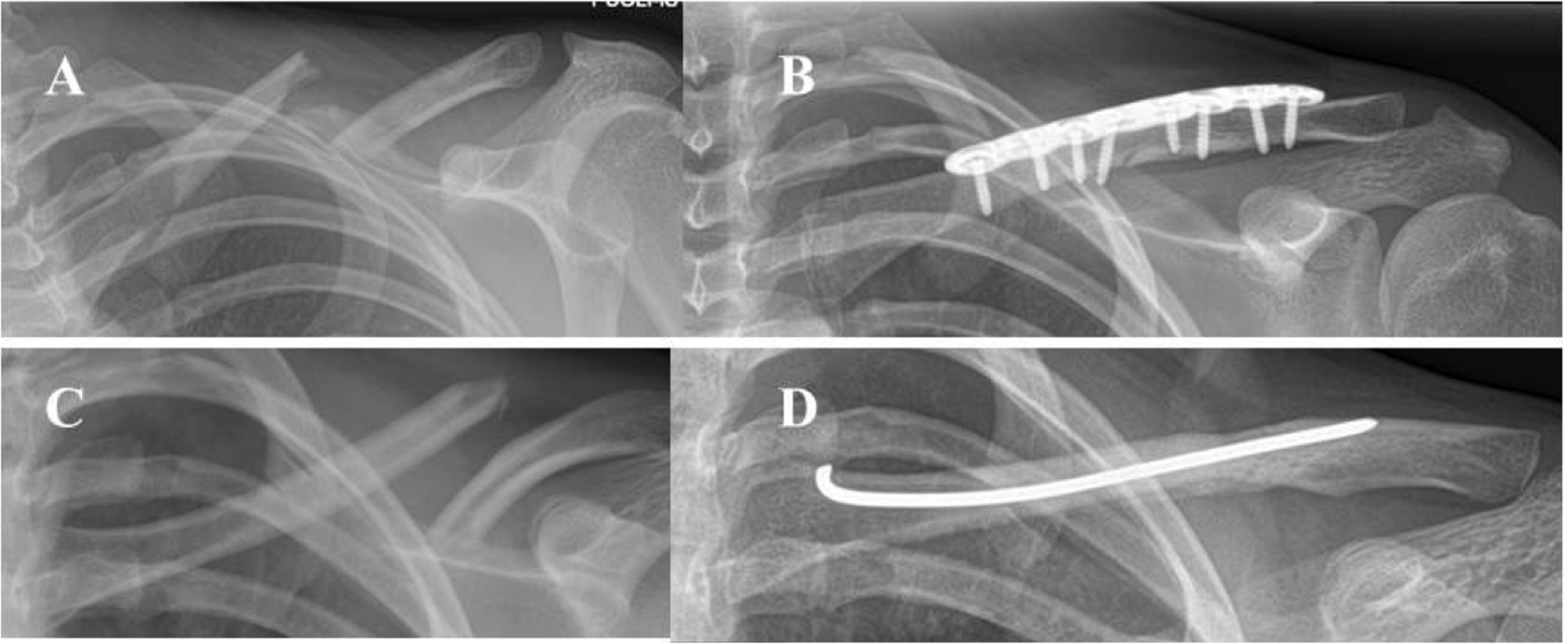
The figure presents the essential surgical methods used in treating middle-third-clavicle fractures by operative means in this study population: Case 1: Preoperative (A) and postoperative (B) radiographs of a patient operated with plate and screw fixation. Case 2: Preoperative (C) radiographs of a patient treated with intramedullary rodding and the respective postoperative radiography (D)
